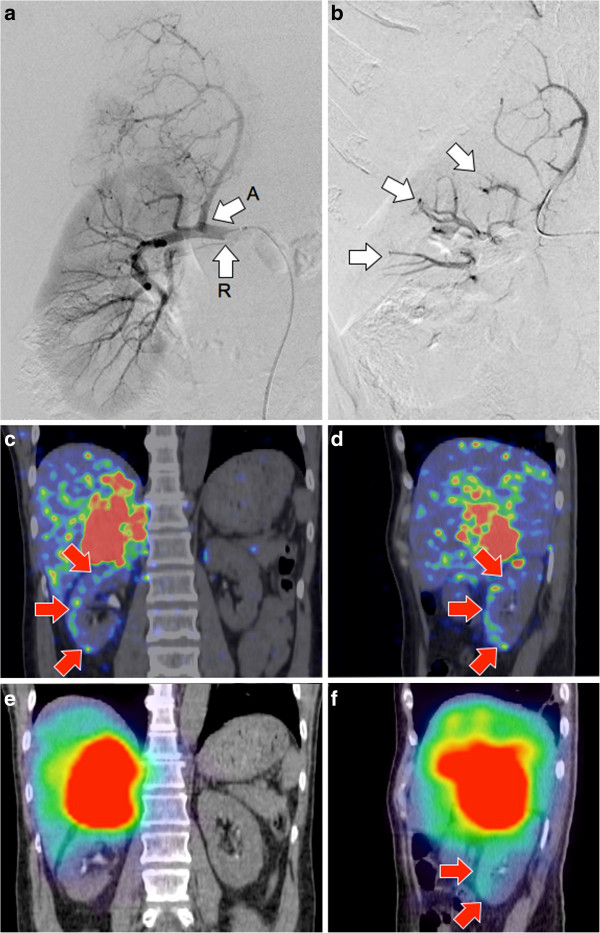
Patient 9.^90 ^Y radioembolization of an organ other than the liver. KIT-negative GIST with bulky metastasis to the right adrenal gland, refractory to tyrosine kinase inhibitors. (a) Pre-radioembolization digital subtraction angiogram (DSA) with catheter tip in the right renal artery ‘R’ demonstrates the origin of the right inferior adrenal artery ‘A’ and the arterial trees of the adrenal tumor and right kidney. The catheter tip was advanced deep into the right inferior adrenal artery for ^90^Y radioembolization. (b) Post-radioembolization DSA with no change in catheter tip position demonstrates significant vascular stasis and reflux of contrast down the right inferior adrenal artery and distally into the terminal branches of the right renal artery (arrows). (c, d)^90^Y PET/CT depicts in high resolution, non-target activity conforming to the anatomy of the right renal cortex (arrows). (e, f) On ^90^Y bremsstrahlung SPECT/CT, non-target activity was indistinguishable from adjacent activity bloom in the coronal plane, but seen as low-grade, diffuse activity in the saggital plane (arrows).
